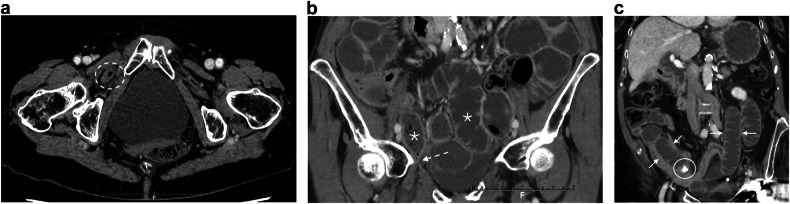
Small bowel obstructions due to causes specifically encountered in the elderly. Obturator hernia in an 85-year-old woman. Axial portal phase CT (a) shows an intestinal segment between the obturator externus and pectineus muscles (dotted circle). Coronal portal phase CT (b) shows distension of the small bowel (asterisk) up to a transition point corresponding to the collar of the hernia (arrow), suggesting a small bowel strangulation due to an obturator hernia. Biliary ileus in a 90-year-old woman. Coronal portal phase CT (c) shows distension of the small bowel (arrows) above a radio-opaque gallstone (circle)
